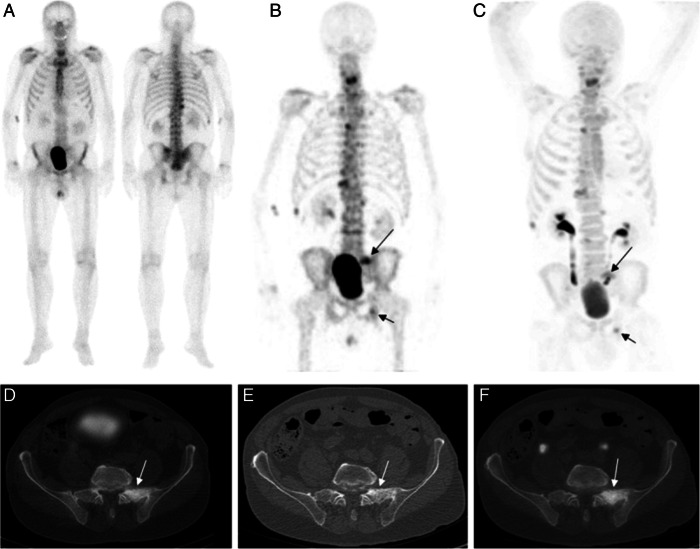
a–f According to one study, no statistically significant systematic differences were observed between results produced using planar whole-body bone scans, SPECT-CT, and PET-CT on newly diagnosed, high-risk prostate cancer (Fonager et al. 2017). But planar whole-body bone scans can lead to misclassifications. Fonager et al. described the following: ‘Anterior and posterior projections of the bone scan (a) were interpreted as non-metastatic on the dichotomous scale (with equivocal uptake in the pelvic region noted on the three-point scale). Both SPECT (b) and NaF PET (c) showed metastatic lesions on the maximum intensity projection images (long and short black arrows).’ (Images courtesy of e-Century PublishingCorporation, Wisconsin, USA)
